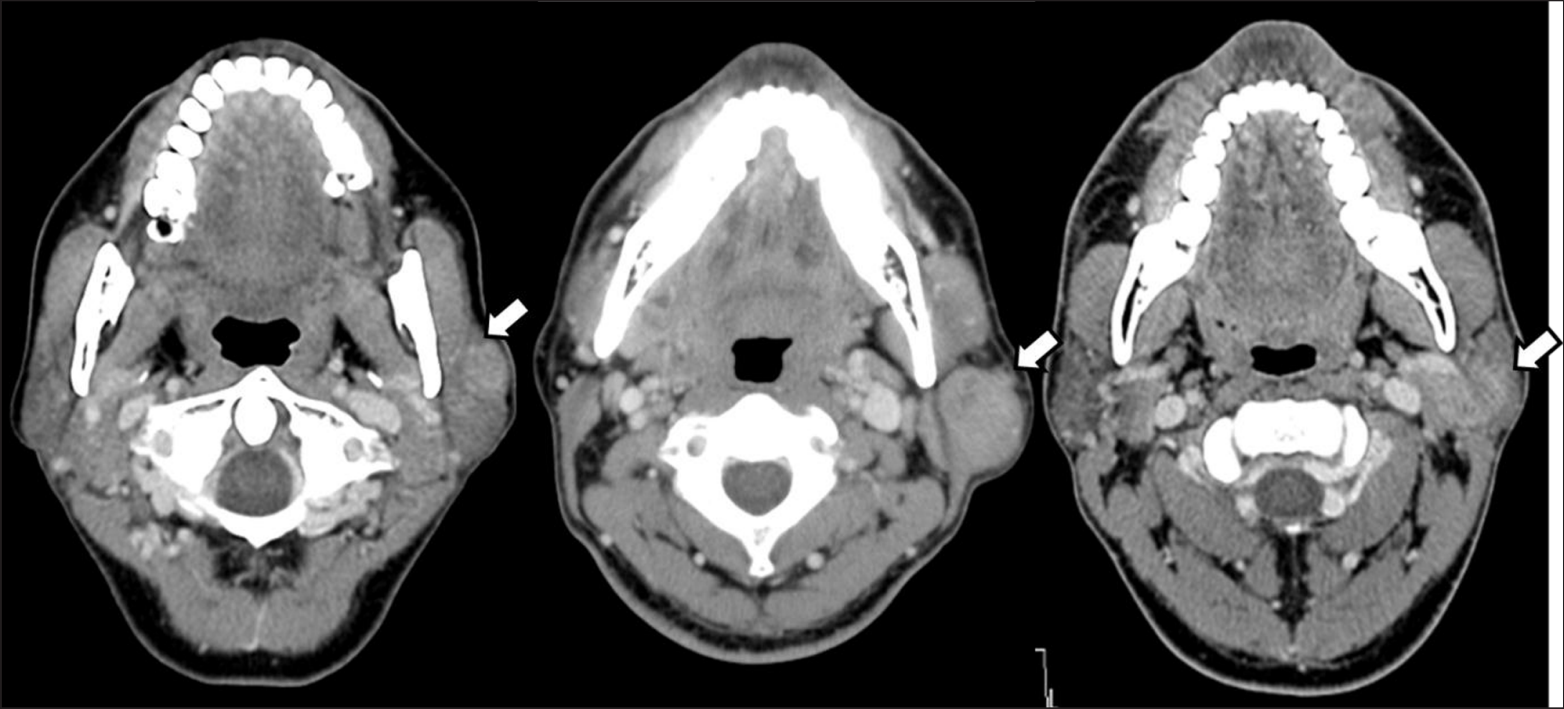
Contrast-enhanced CT images of parotid gland tumors (arrows) in three different patients (the left, 49-year-old woman; the center, 45-year-old man; the right, 68-year-old man) obtained 70 seconds after beginning of contrast infusion. As compared with a 50-second scan delay, Warthin tumor (center) does not show strong enhancement, a characteristic finding to suggest rich microvessels, it is therefore hardly distinguished from other tumors (pleomorphic adenoma [left] and acinic cell carcinoma [right]).
